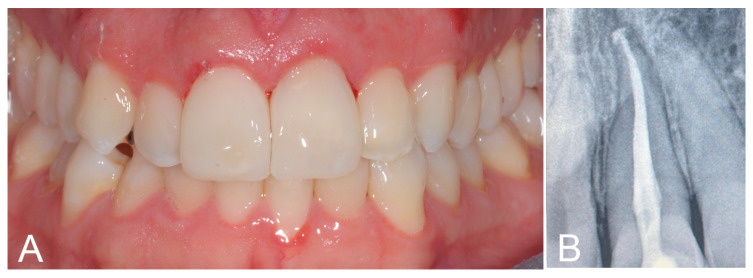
(A) Final esthetic result. (B) Radiographic check for the absence of any excess material in the cervical area.
